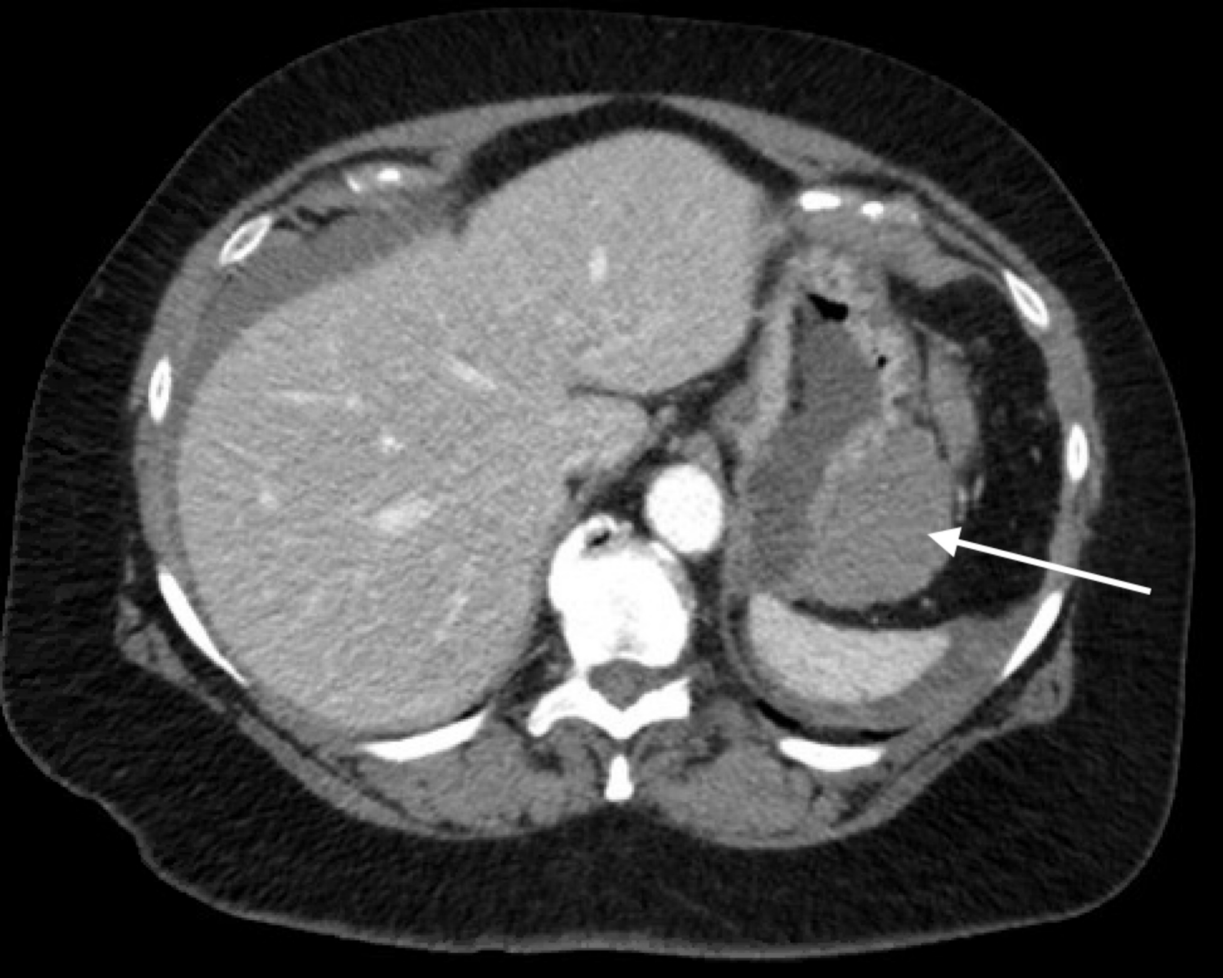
Axial view of the CT scan showing hemoperitoneum around the upper abdominal viscera and adjacent to the stomach (arrow)
